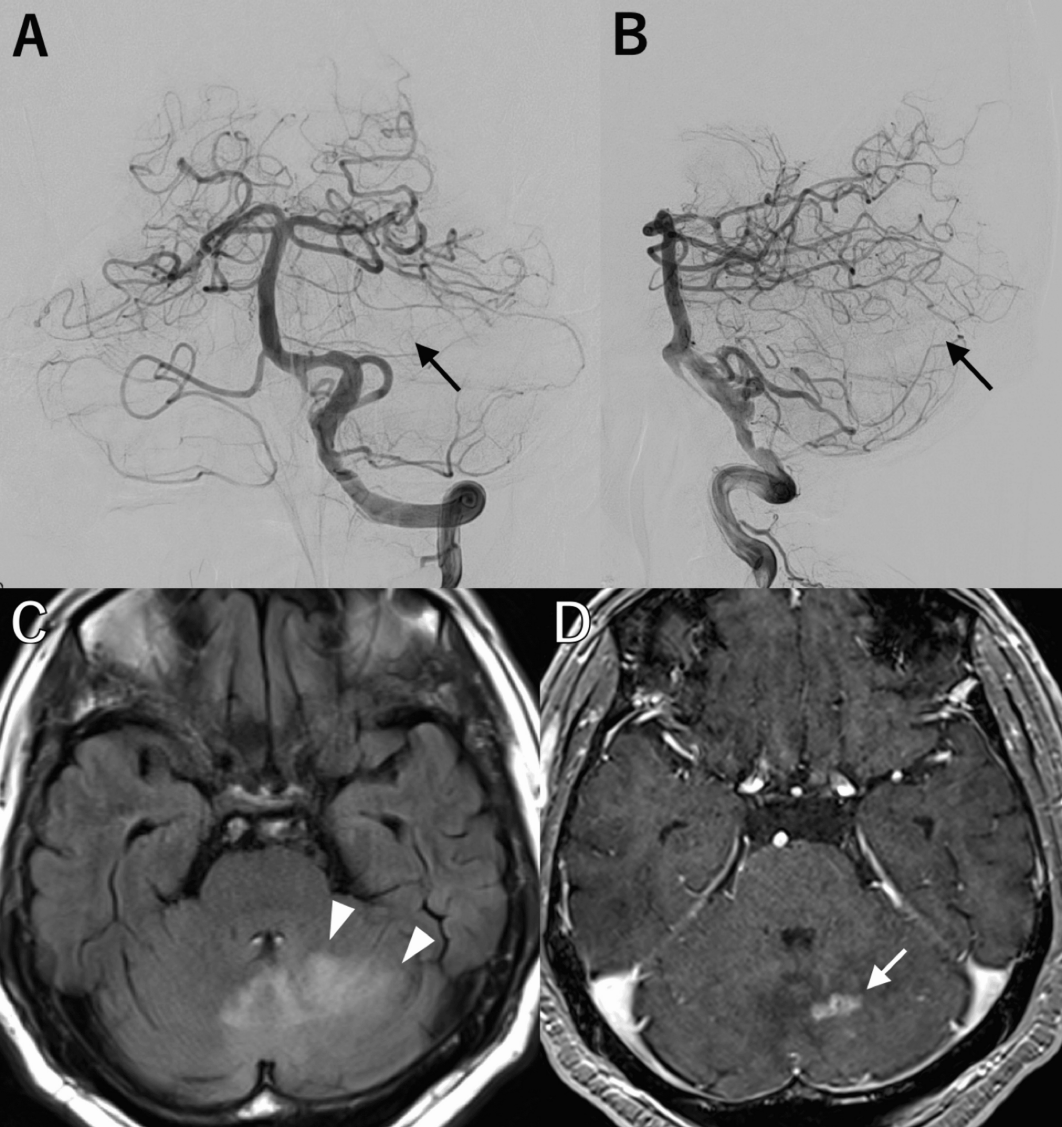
Images at six months after stereotactic radiosurgery. A, B: Catheter angiograms of the left vertebral artery, shown in frontal view (A) and lateral view (B), showing complete obliteration of the arteriovenous malformation (AVM). C, D: Magnetic resonance imaging demonstrating asymptomatic surrounding edema in the right cerebellar hemisphere on fluid-attenuated inversion recovery imaging (C, arrowheads) and a gadolinium-enhanced lesion at the prior AVM site on T1-weighted imaging (D, arrow).
